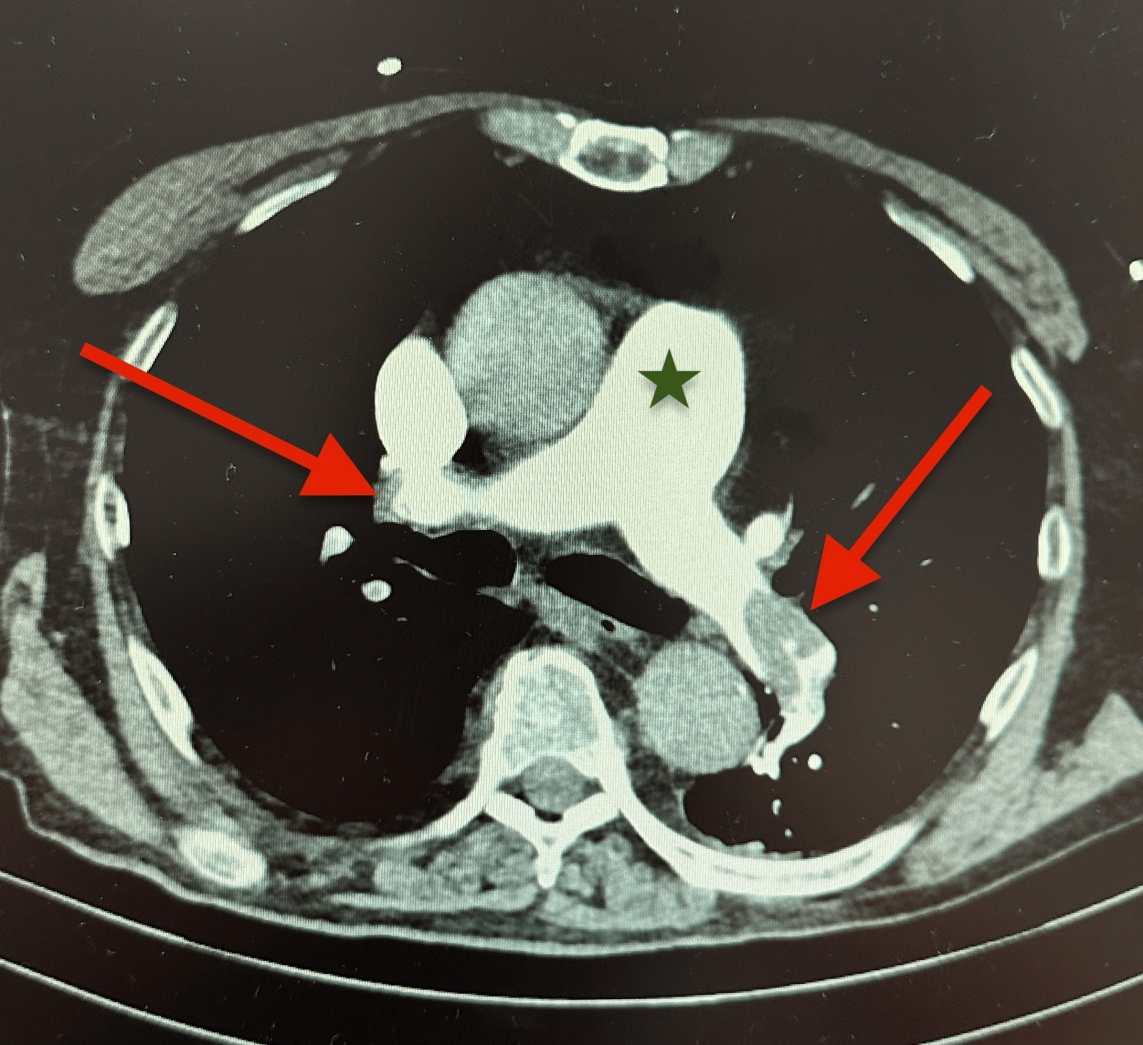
Computed tomography pulmonary angiography demonstrating acute pulmonary embolism. Axial computed tomography pulmonary angiography (CTPA) showing extensive intraluminal filling defects within both main pulmonary arteries (arrows) and dilation of the main pulmonary artery, consistent with acute pressure overload in high-risk pulmonary embolism.
